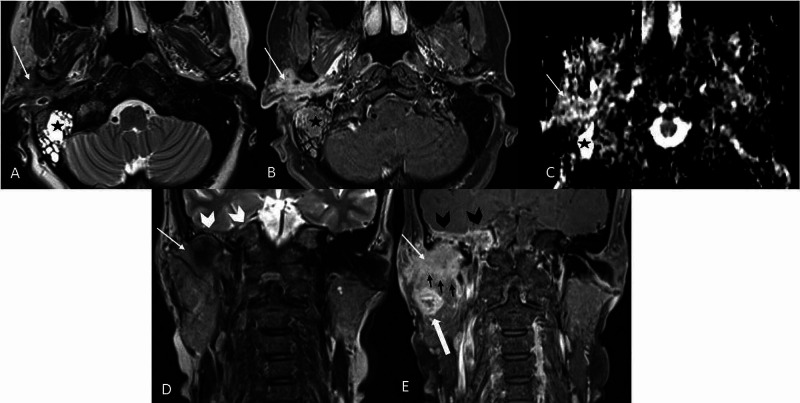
MRI of the base of skull and neck in (A) axial T2-weighted image, (B) axial T1 post-contrast with fat suppression, (C) ADC map, (D) coronal T2-weighted image, and (E) coronal T1 post-contrast with fat suppression. The lesion is hypointense on T2, enhances homogeneously, and shows mix low and high signals on ADC (white arrow). Loss of the hypointense bony cortex of the petrous apex and the floor of the middle cranial fossa (white arrowhead) results in extension of the lesion/inflammation to the right petrous apex; note the involvement of the dural reflection at the floor of the right middle cranial fossa (black arrowhead). The lesion infiltrates the parotid gland inferiorly (black arrow). Intraparotid necrotic node is present (block white arrow). The lesion demonstrates an infiltrative pattern, lacking a significant mass effect. Retention fluid occupies the right mastoid air cells (black star). MRI, magnetic resonance imaging; ADC, apparent diffusion coefficient
